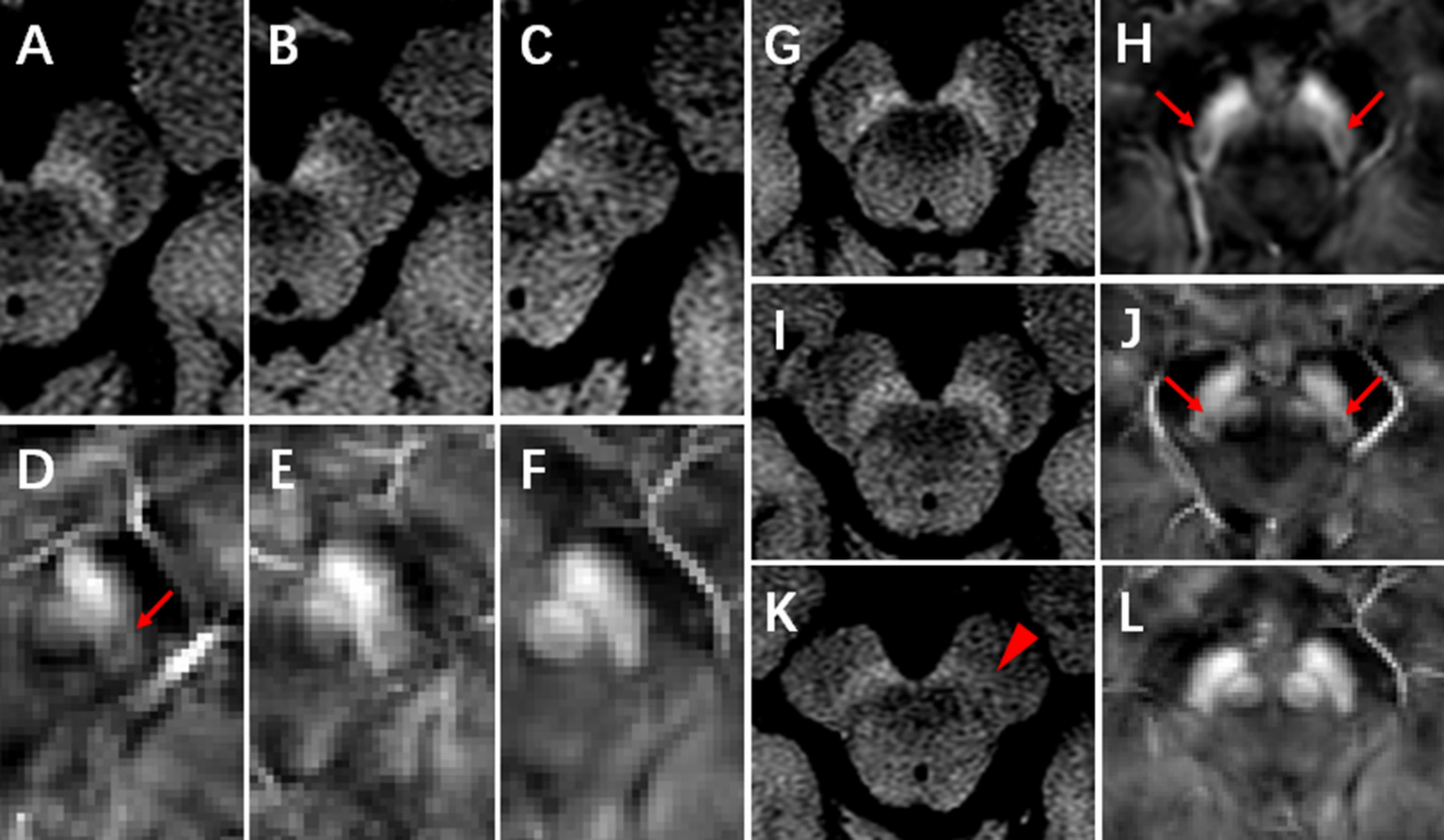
NM-MRI and QSM images. (A–C) represent the normal, possibly abnormal, and definitely abnormal SN on NM-MRI, respectively. Nigrosome-1 (N1) could be visualized in the dorsal part of the healthy SN on QSM images (D, arrow). (D–F) represent that N1 was present, indecisively present and absent, respectively. (G,H), a control subject, female, 65 years, neuromelanin was normal (G) and N1 was present (H, arrow) in bilateral SN. (I,J), an ET patient, 59 years, female, neuromelanin was normal (I) and N1 was present (J, arrow) in bilateral SN. (K,L), a *de novo* PD patient, 75 years, female, neuromelanin was definitely abnormal in unilateral SN (K, arrowhead) and N1 was absent in bilateral SN (L).
